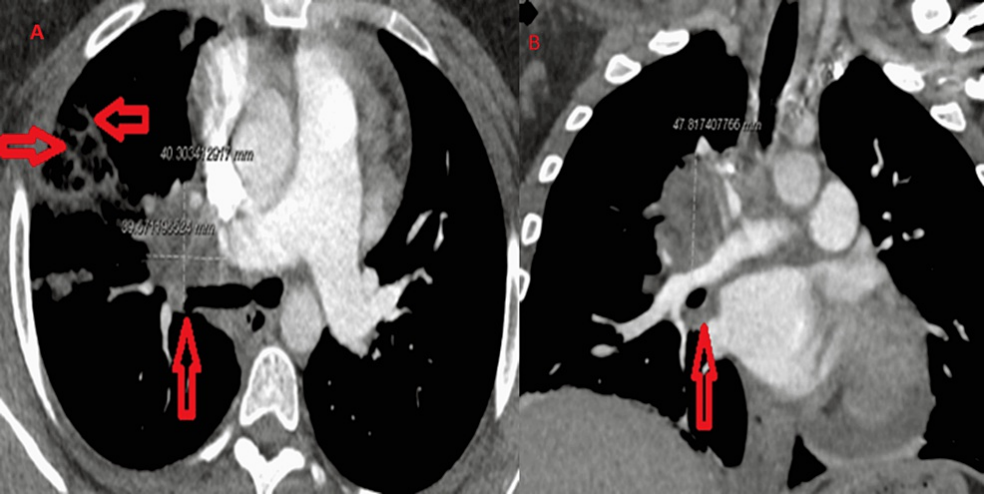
CT chest showing a right hilar mass (vertical red arrow in axial image 2A) with multifocal opacities in RUL (horizontal red arrows axial image 2A) encasing the right upper lobe bronchus and the right upper lobe’s pulmonary artery causing luminal narrowing with post-obstructive atelectasis (vertical arrow coronal image 2B) RUL: right upper lobe
